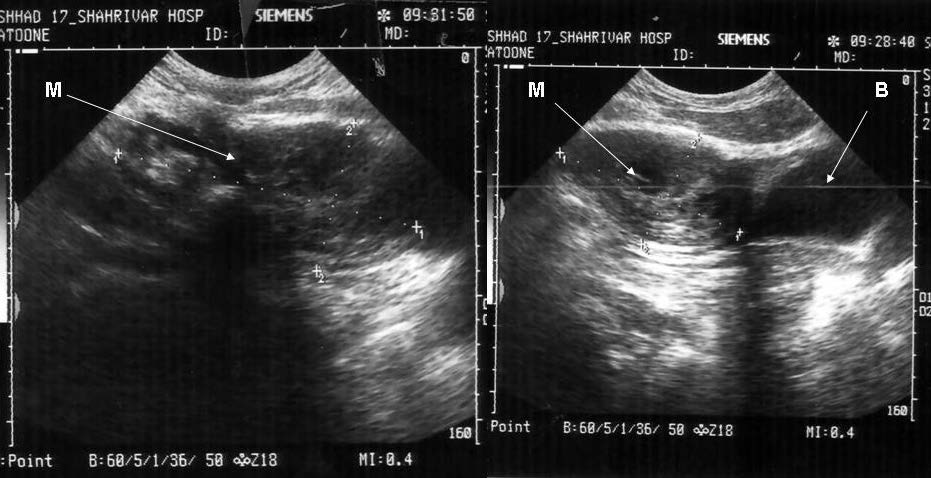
Heterogeneous Mass in the Sonographic Examination M: Mass, B: Bladder
